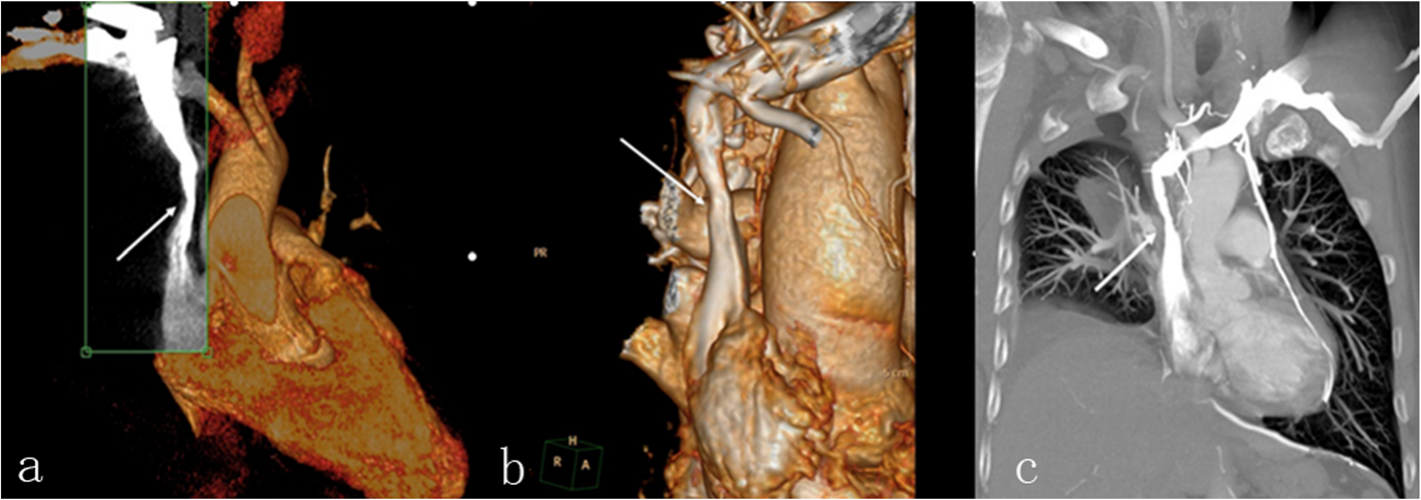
a and b Postoperative thoracic spiral computed tomography (CT) plus 3D reconstruction showing unobstructed, reconstructed SVC with adequate filling (*white arrow*). c CT venogram showing adequate postoperative filling of reconstructed SVC (*white arrow*)
